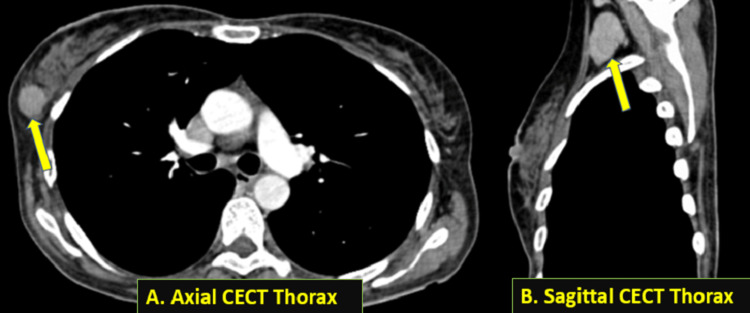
(A) Axial section, arterial phase-contrast enhanced computed tomography (CECT) image (soft tissue window) showing an enhancing mass lesion (shown by the arrow) in the upper outer quadrant of the right breast. (B) Sagittal section, venous phase-contrast enhanced computed tomography (CECT) image (soft tissue window) showing a heterogeneously enhanced enlarged lymph node (shown by the arrow) in the right axilla.
